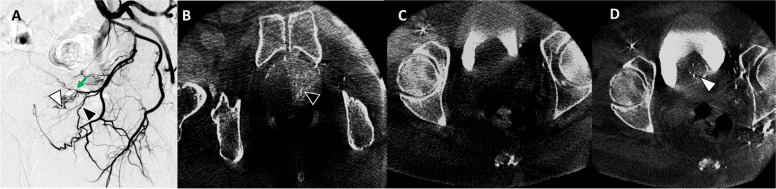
A Left internal iliac artery angiogram demonstrated prostatic artery (black arrowhead) arising off the left obturator artery. B After the successful left side embolization, then the right side was embolized. C CBCT without contrast injection at completion was attempted to ensure adequate coverage. This did not show coverage for the median lobe. D Then further review showed an accessory prostatic artery from the left superior vesical artery (green arrow). The branch of superior vesical artery (white arrowhead in panel A) then showed perfusion of the median lobe which was confirmed on CBCT (white arrowhead)
